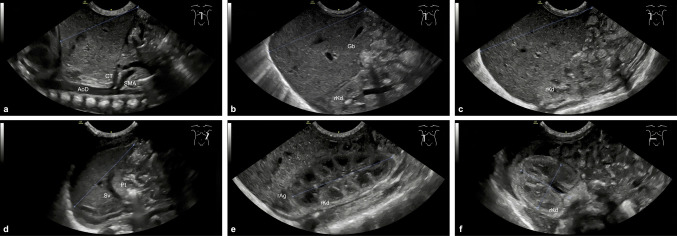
Standardized ultrasound planes for abdominal organ measurement. a: liver length measured in the midsternal line. b: liver length measured in the midclavicular line. c: liver length measured in the anterior axillary line. d: splenic length measured in a longitudinal oblique scan. e: right kidney imaged longitudinally from a frontal approach. f: right kidney imaged in transverse plane from a frontal approach. AoD: descending aorta, CT: coeliac trunk, Gb: gallbladder, Pt: pancreatic tail, rAg: right adrenal gland, rKd: right kidney, SMA: superior mesenteric artery, Sv: splenic vein
